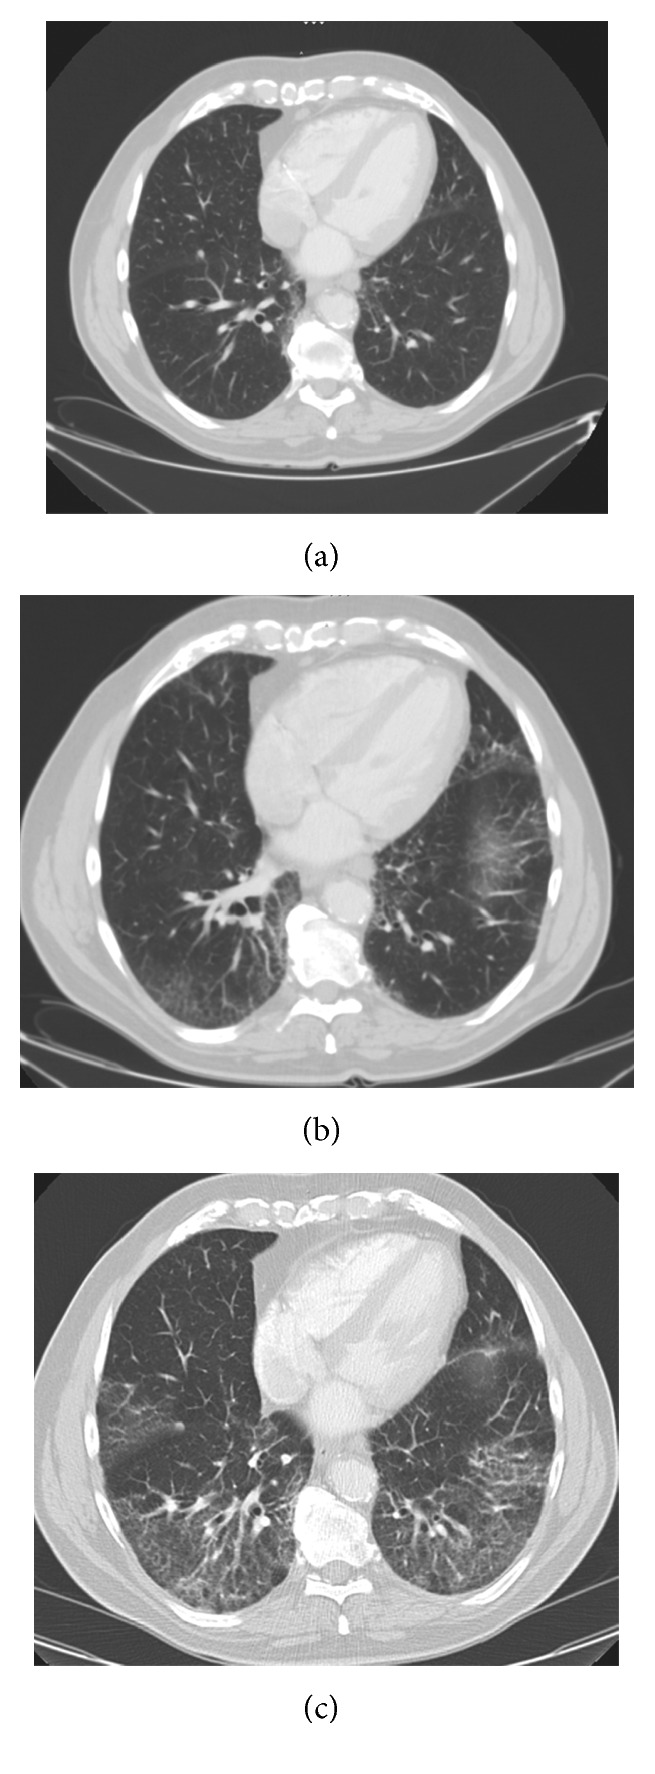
Computed tomography findings. (a) January 2015: the lower lung shows normal lung structures without signs of interstitial pneumonia or fibrosis. (b) June 2015: the same area now shows a bilateral mild interstitial pneumonia with ground-glass opacities. (c) March 2016: images show a more fibrotic structure with mild reticular abnormalities and ground-glass opacities.
